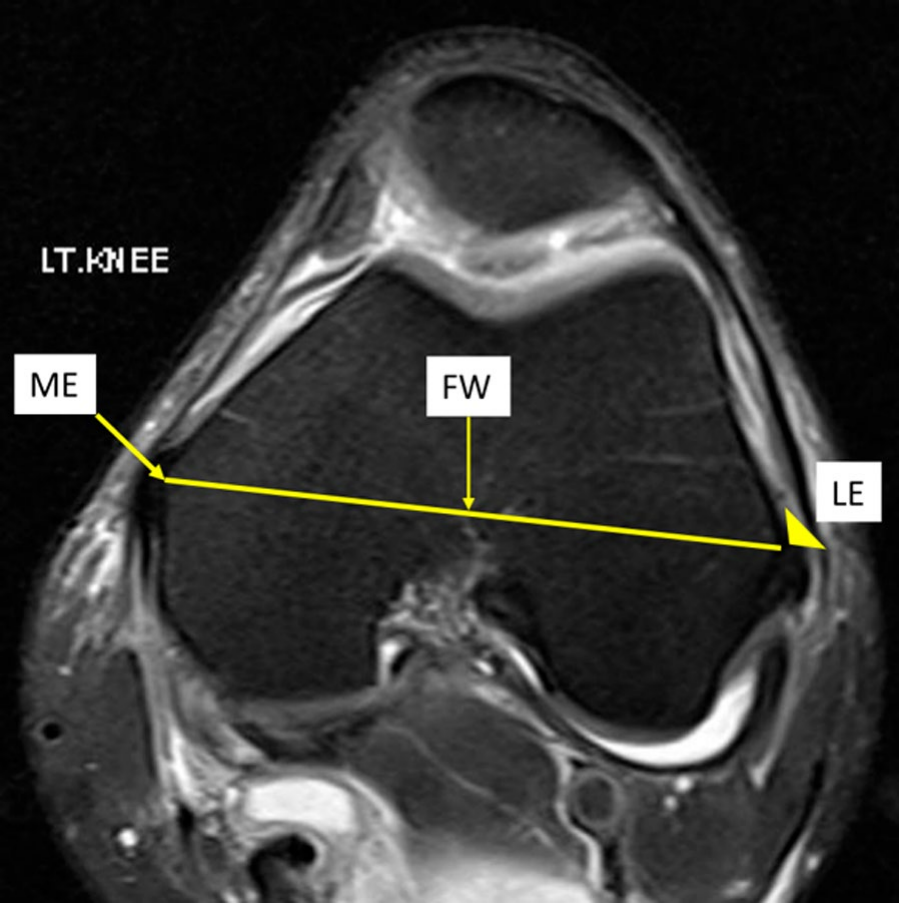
Demonstration of the measurement technique in axial view of MRI. The femoral transepicondylar width (FW) is the distance from the most prominent point of medial femoral epicondyle (ME, arrow) to the most prominent point of the lateral femoral epicondyle (LE, arrowhead)
